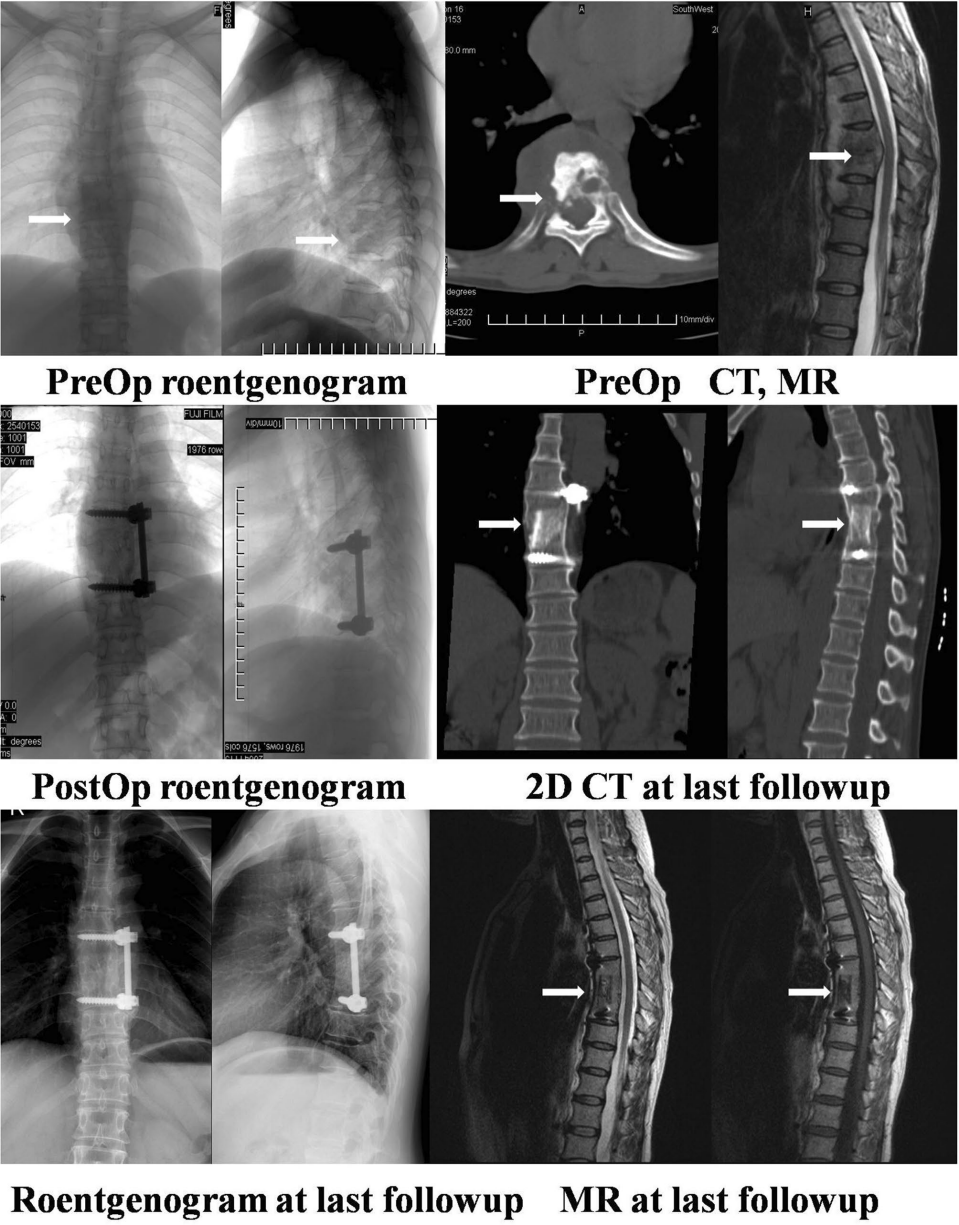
A 35-year-old woman complained of severe back pain and lower extremity weakness. Preoperative radiographs, CT scans and MRI scans showed T8/9 disc space with the destruction of adjacent vertebral bodies and paravertebral abscess with spinal cord compression (white arrows). The kyphotic angle was 15°. The anteroposterior and lateral roentgenograms taken after surgery showed the long segment tricortical iliac crest allograft inserted from T7–T9. The CDH rod was placed for added stability to prevent graft displacement. The kyphotic angle improved to 9°. The 2D CT scan taken at the 10-year follow-up showed that the graft integrated with the adjacent vertebral bodies. The roentgenogram showed solid bony fusion, and the loss of correction was 3°. The MR scan taken at the final follow-up showed healed vertebrae and no compression of the spinal cord.
